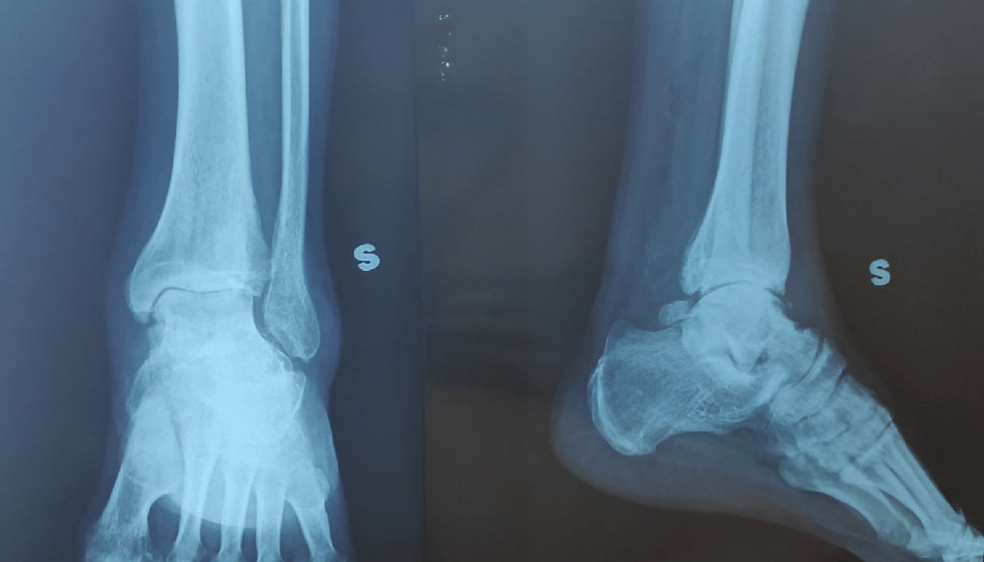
Anteroposterior (AP) and lateral view X-rays of the left ankle show ankle osteoarthritis, tarsal bones, osteoporosis, and talus avascular necrosis (AVN)
